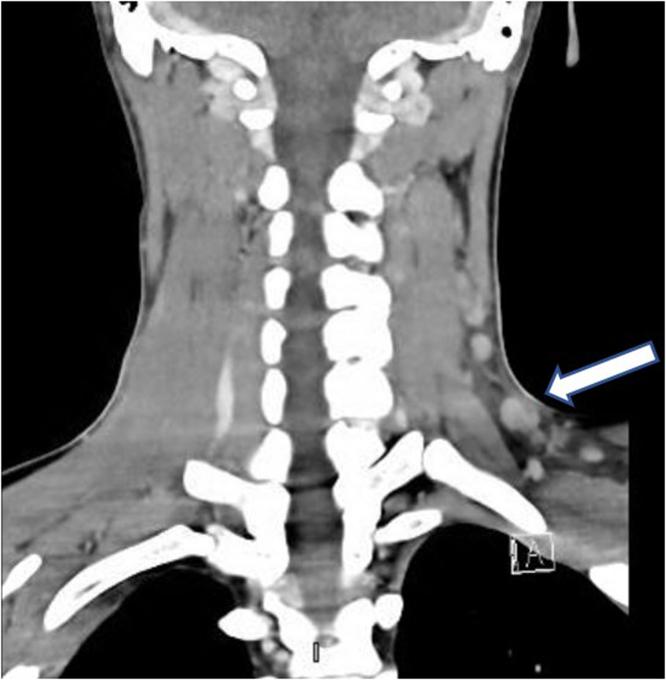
CT of the neck demonstrating left multiples supraclavicular lymphadenopathy the largest measured 10 × 11 mm (arrowed).
